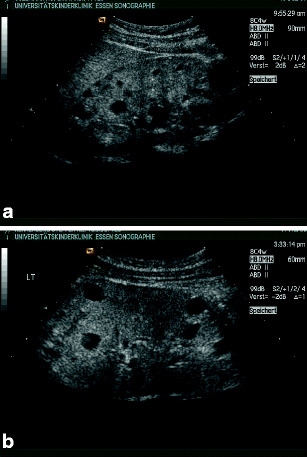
Typical sonographic image of autosomal dominant polycystic kidney disease (ADPKD): a 10-year-old girl (father ADPKD); b 11-year-old boy (father ADPKD)
